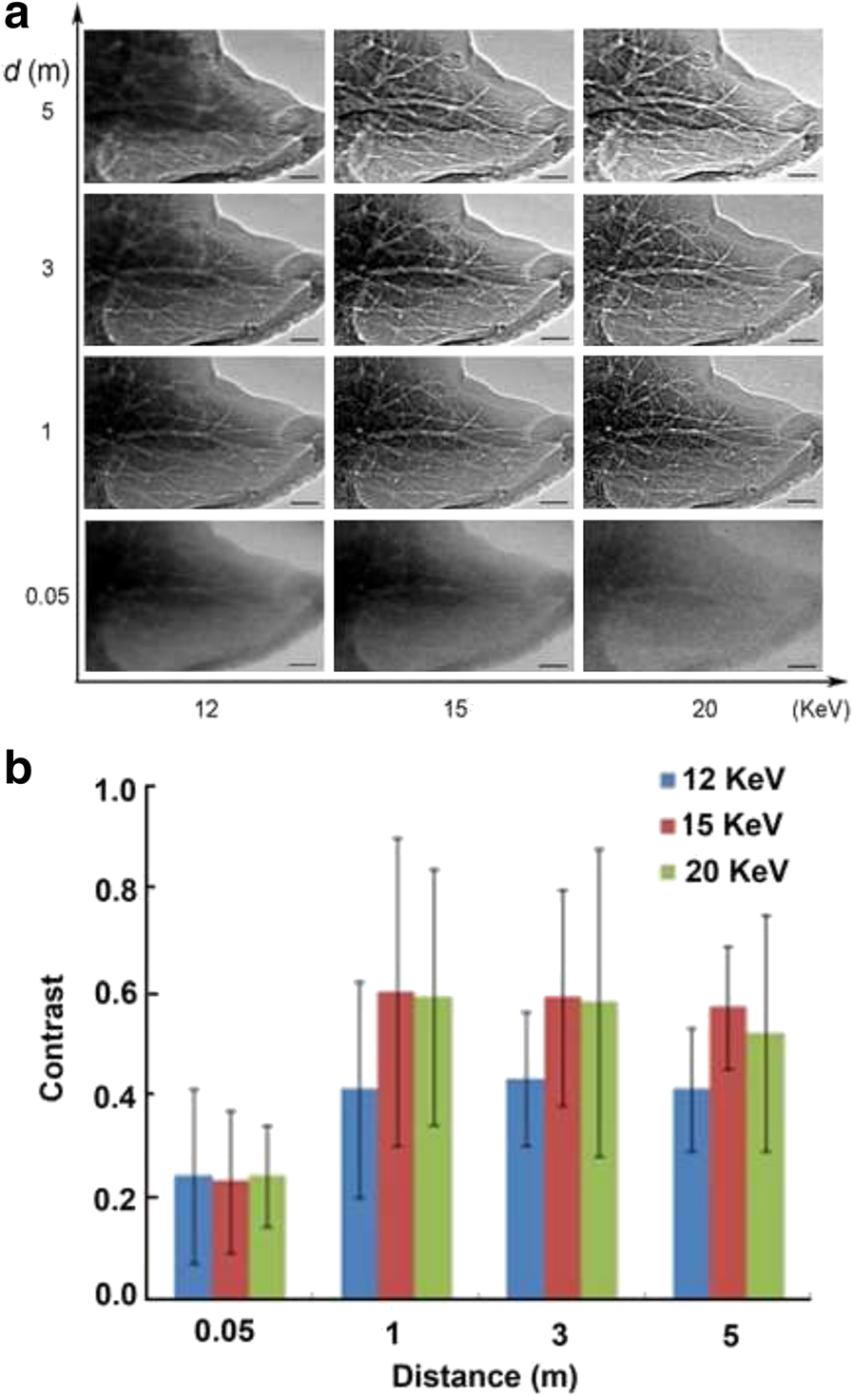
Two-dimensional projection images vs. hepatic vascular phase contrast at different X-ray energies and object-to-detector distances. a Normal liver lobe with X-rays set to 12, 15 and 20 keV. Each energy condition was used at 0.05, 1, 3 and 5 m. Bar = 1 mm. b Quantitative comparison of image contrast at different X-ray energy levels and object-to-detector distances. The best image contrast was obtained using 15 keV and at 1 m
